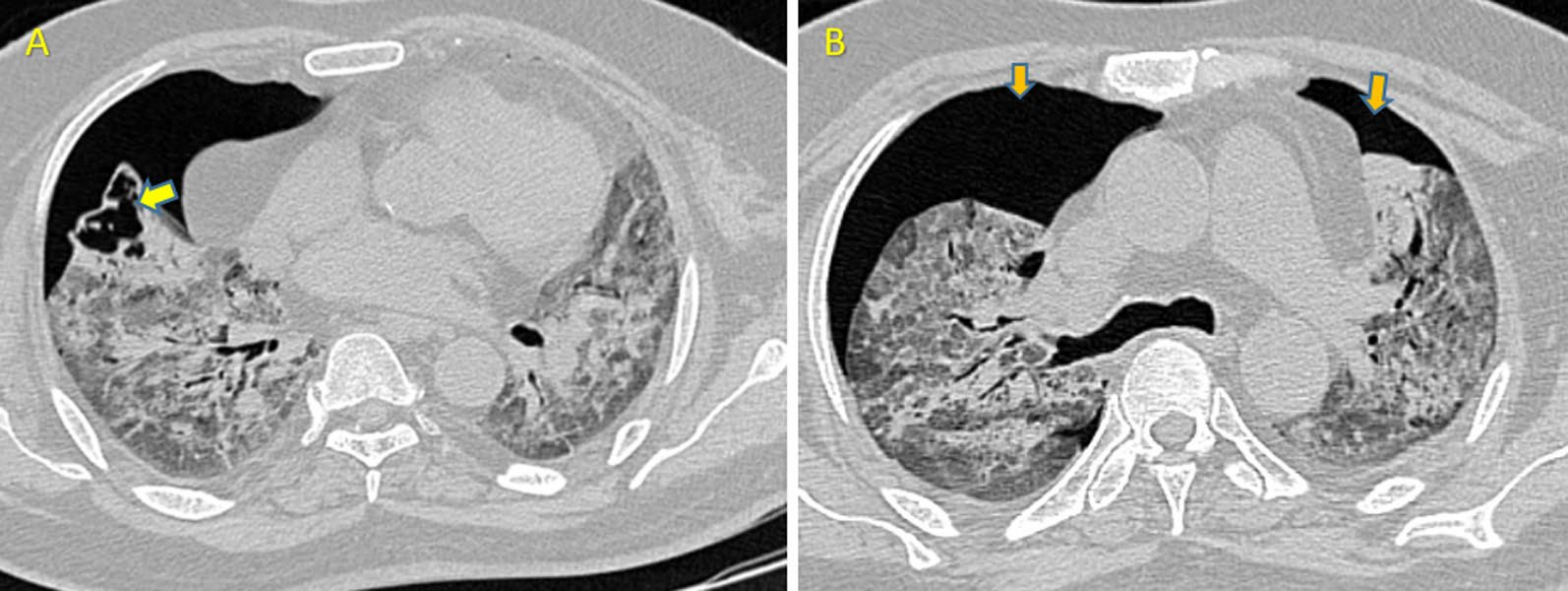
Axial HRCT scans showing imaging features of Candidiasis in case 5 of COVID-19. A Axial HRCT thorax of case 5 shows small cavitary lesions with few internal septations and tiny soft tissue nodular opacities within (yellow arrow in A). Sputum culture revealed Candida species. B Bilateral pneumothorax can be seen (orange arrows in B)
